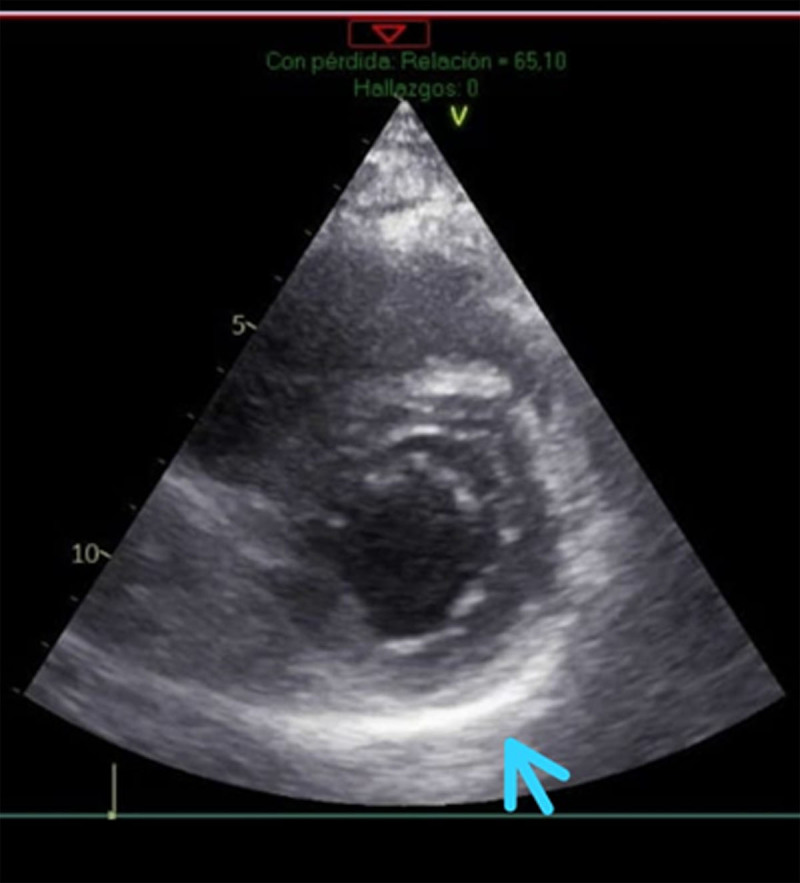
Echocardiogram of the short axis with thickening of the parietal pericardium.
